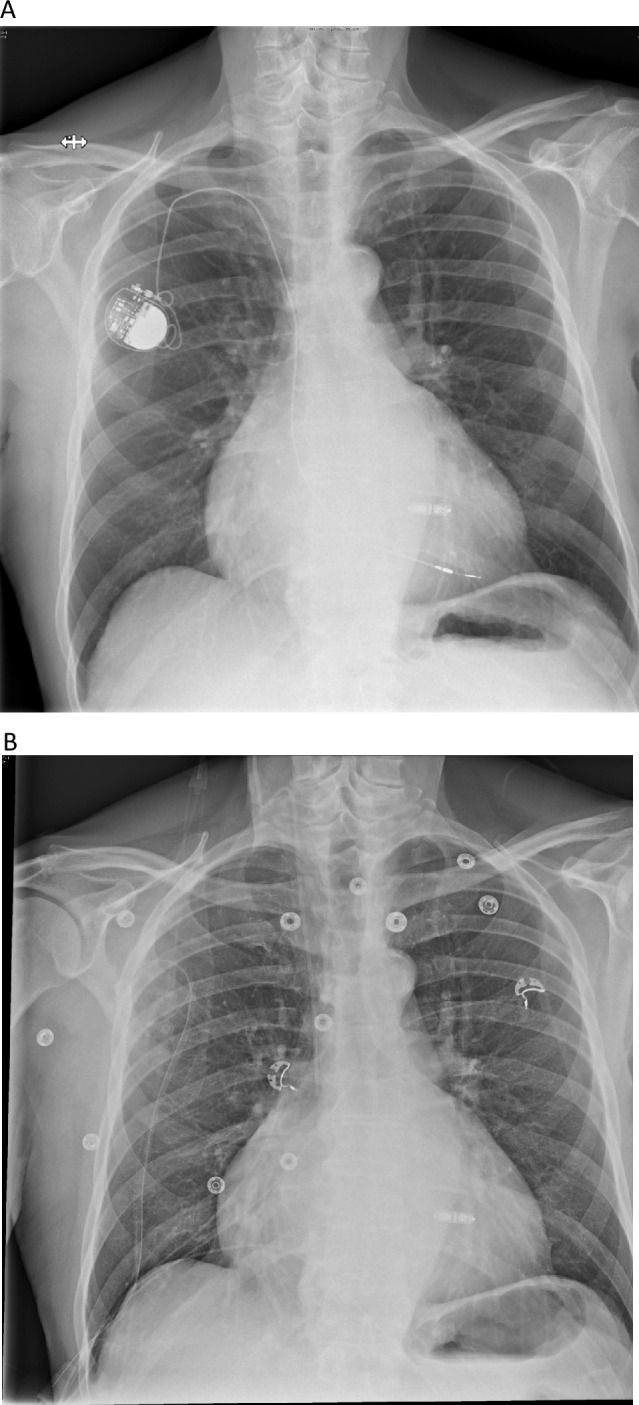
A Chest X-ray after MICRA Implantation and prior to lead extraction. B Chest X-ray after lead extraction showing stable position and no dislocation of the leadless system
